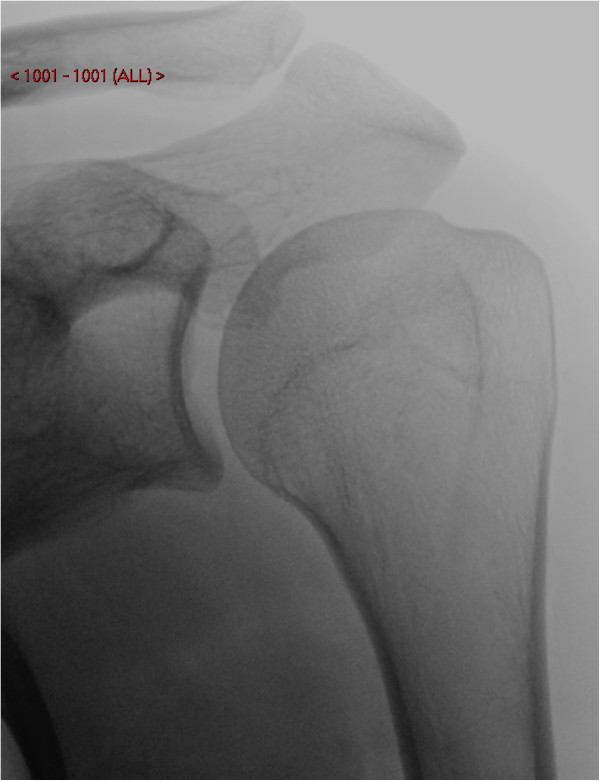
Anterior-posterior radiograph of the left shoulder.
